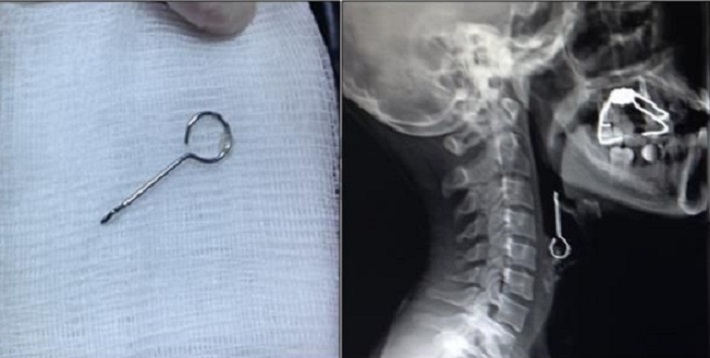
X-Ray and foreign body's of Case 2
